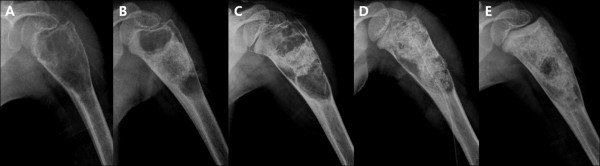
Insufficient DBM in quantity leaded to inadequate healing of the cyst. Plain radiographs A) of the proximal humerus in a 3-year-old boy B) at postoperative 3 months showing filling defect of DBM C) at postoperative 14 month showing recurrence of cyst D) after repeat intervention E) at 13 additional months of follow-up after a second intervention.
